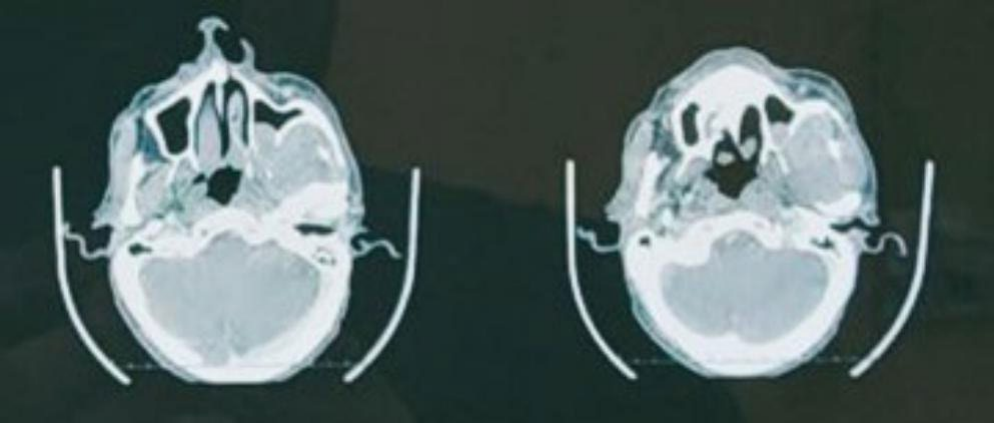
CT imaging in axial section showing the extension of lesion into left infratemporal region with erosion of pterygoid plates
